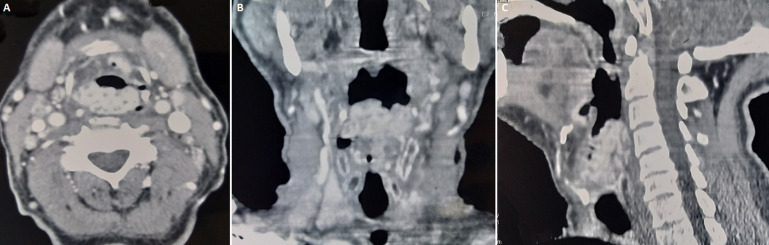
axial (A), coronal (B) and sagittal (C) CT scan images showing a large mass arising from the false vocal cord and extending to supra and subglottis
